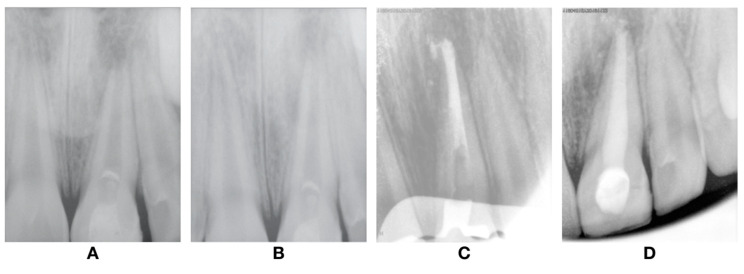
Follow-up radiographs after revascularization. (A) 1-year follow-up; (B) 4-year follow-up; and after (C) plug MTA; and (D) root canal obturation.
